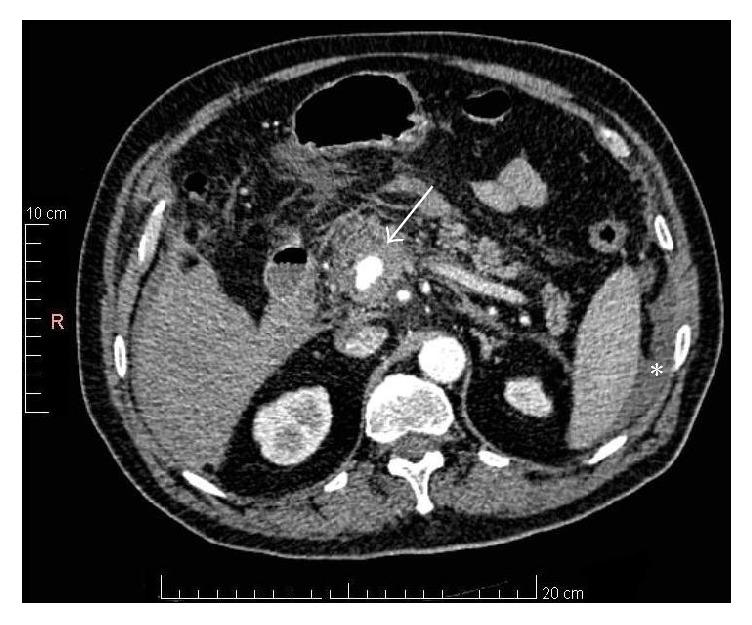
Contrast-enhanced CT scan of the abdomen prior to transarterial embolization*: A 2.0- × 1.4 cm visceral aneurysm* (Arrow)* surrounded by 0.7 cm rim of thrombus arising from the inferior pancreaticoduodenal branch. The hemoperitoneum is also noticed in this figure* (Asterisk)*.
